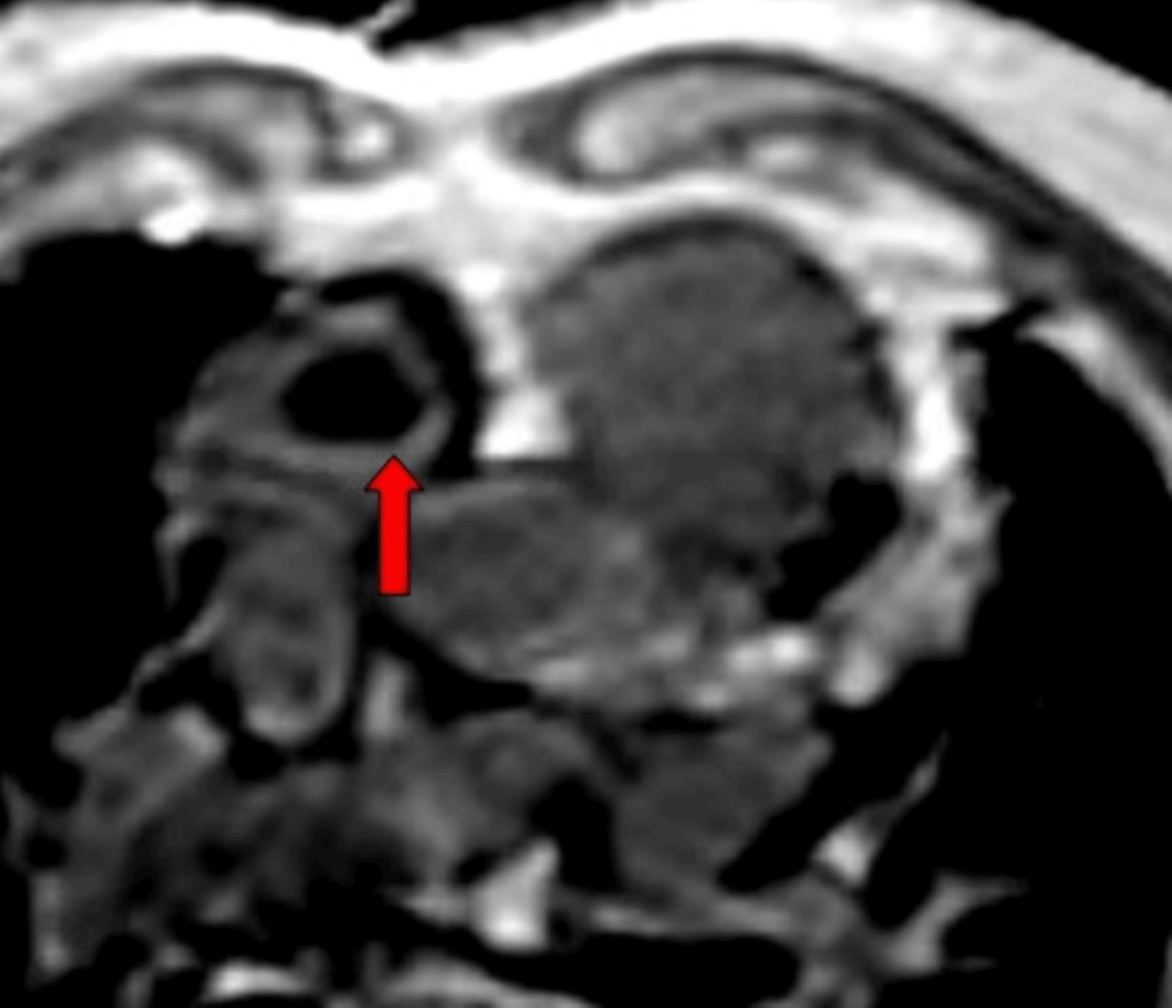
Cardiac magnetic resonance imaging in the four-chamber view shows a 2.1 cm x 1.5 cm mass with no enhancement on a Ti600 sequence consistent with a thrombus
